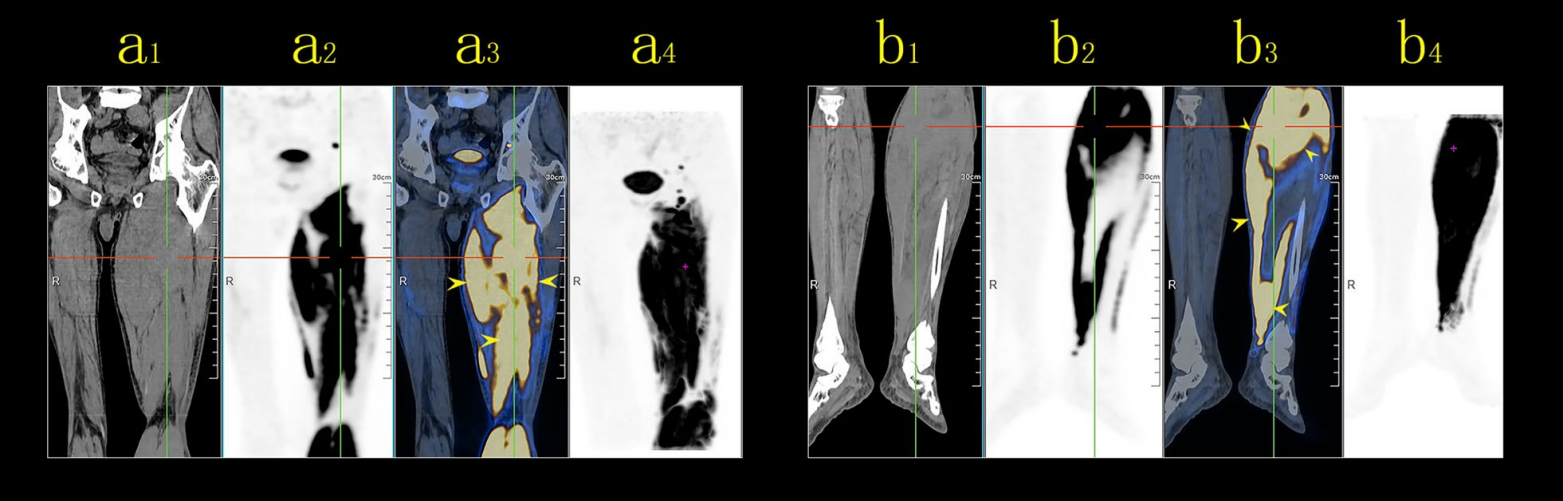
Positron emission tomography/computed tomography (PET/CT) showing multiple lesions of increased FDG-uptake in the left obturator externus muscle, the muscles of the medial and posterior compartments of the thigh (a), and the posterior and peroneal muscles of the calf (b). (a1 &b1) CT image showing ill-defined intramuscular lesions. (a3 & b3) PET/CT image showing multiple lesions with FDG uptake (maximum standardized uptake: 10.20). (a2, a4 and b2, b4) FDG images
